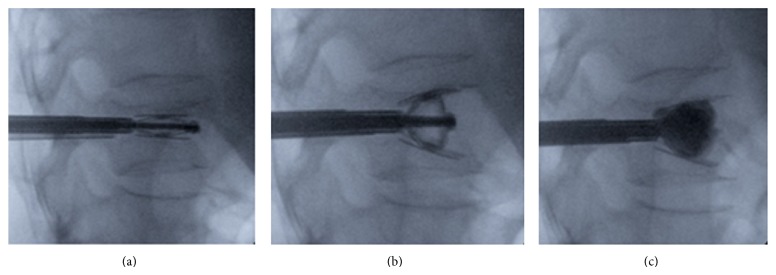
Intraoperative radiographs: (a) expansion of SpineJack implant; (b) fracture reduction; (c) injection of Cohesion bone cement.
